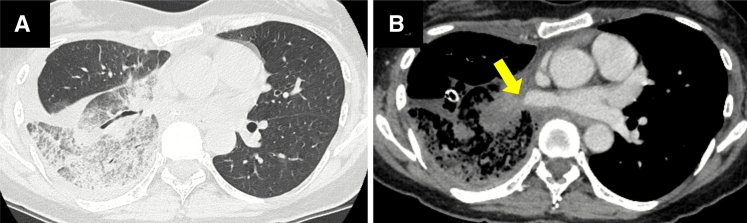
Computed tomography (*CT*) images. A, Localized consolidation in the middle lobe and pleural effusion. B, Contrast CT image showing interruption of the middle lobe pulmonary vein (*arrow*).
